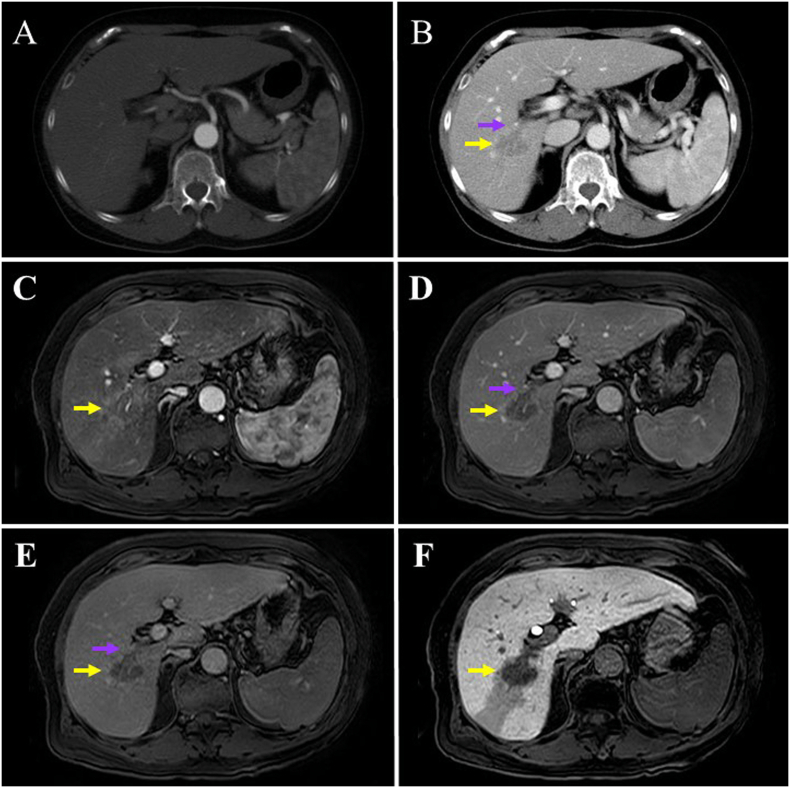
Dynamic computed tomography and magnetic resonance imaging findings. The images show a solitary tumor measuring 3 cm in size (yellow arrows) accompanied by portal vein (PV) thrombosis (suspicious tumor thrombosis) (purple arrows). In dynamic computed tomography, the tumor is enhanced during the arterial phase (A), and only subtly enhanced during the portal phase (B). In dynamic magnetic resonance imaging, the tumor is unenhanced during the arterial phase (C), and only subtly enhanced during the portal (D) and parenchymal (E) phases. The tumor is visible as a clear defect during the parenchymal phase in gadolinium-ethoxybenzyl-diethylenetriamine-pentaacetic acid-enhanced magnetic resonance images (F). (For interpretation of the references to colour in this figure legend, the reader is referred to the web version of this article.)
